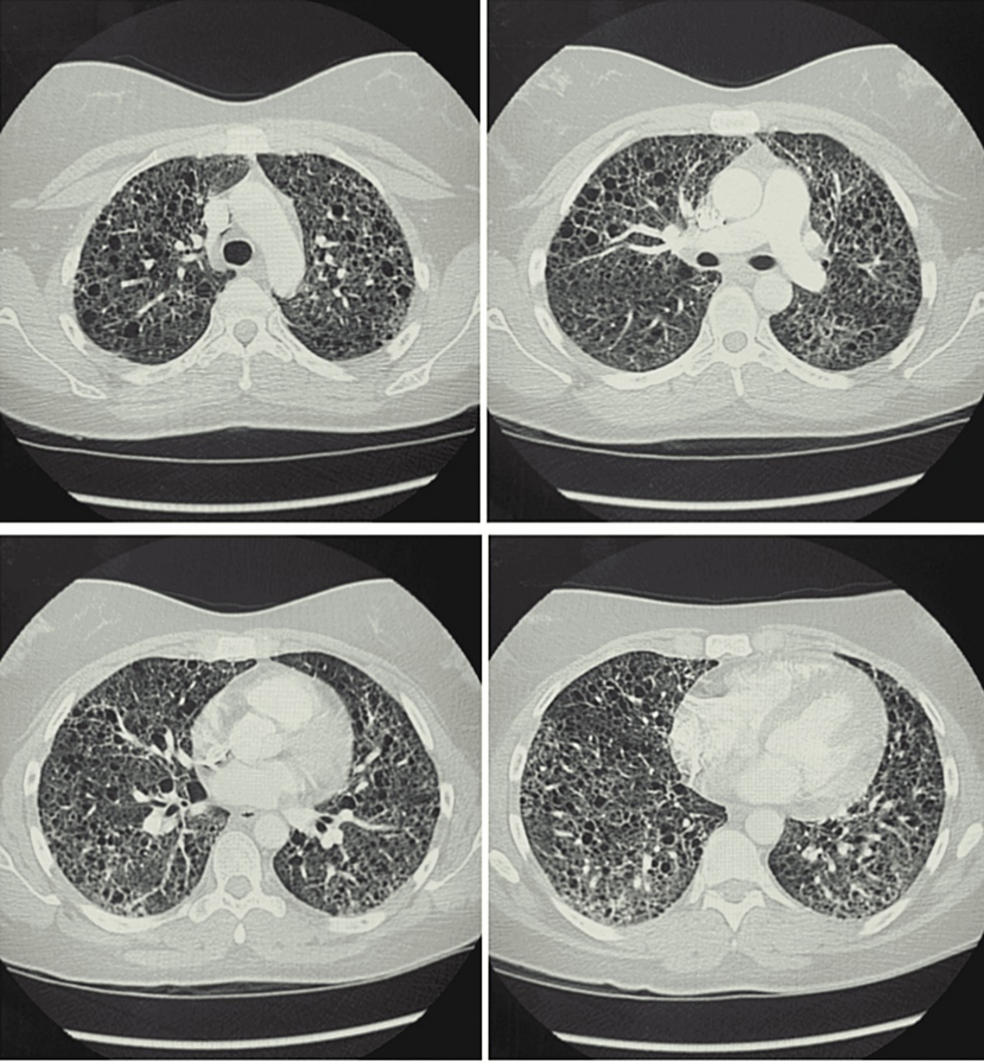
CT chest demonstrating extensive cystic changes throughout the lungs bilaterally and diffuse patchy ground-glass densities, consistent with underlying lymphangioleiomyomatosis and superimposed COVID-19 pneumonia.
